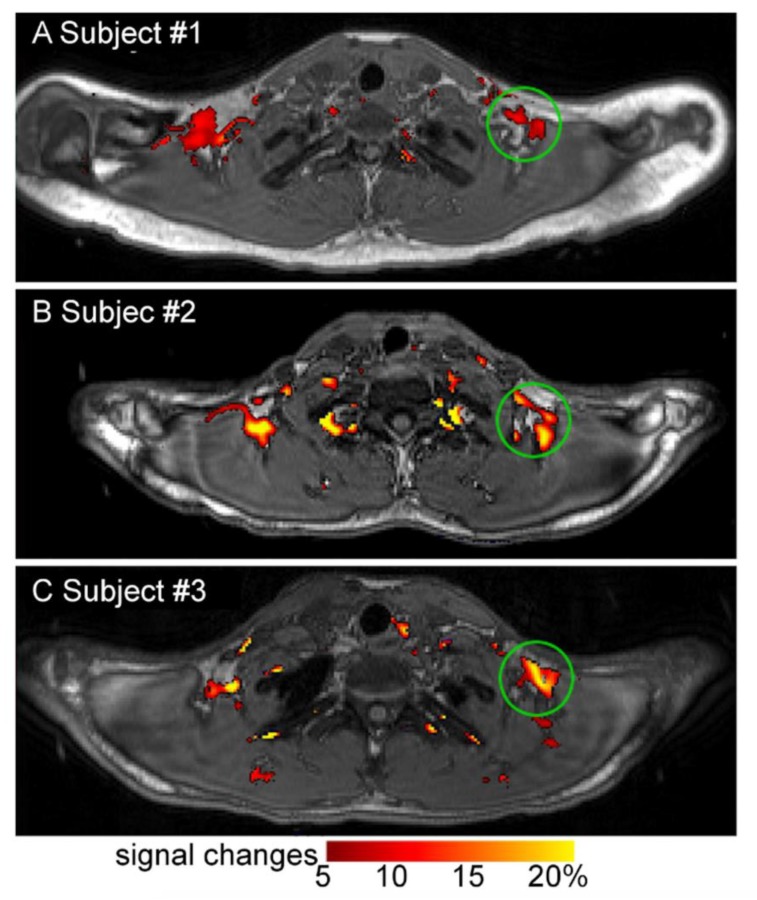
Change in blood oxygen level dependent MRI signal in human BAT with cold exposure. Change in blood oxygen level dependent MRI signal in supraclavicular BAT with cold exposure (13–16 °C) in healthy human research subjects. Images A, B, and C are representative images of 3 subjects. Image reproduced with permission from reference 47.
